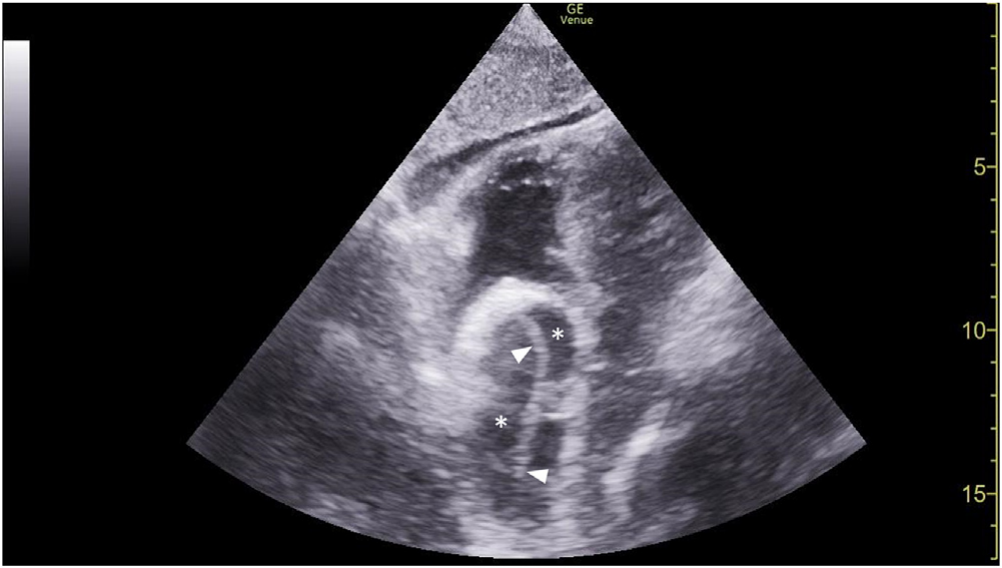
Subxiphoid point-of-care ultrasound showing ascending aorta (asterisks) with an echogenic linear dissection flap (arrowheads).
